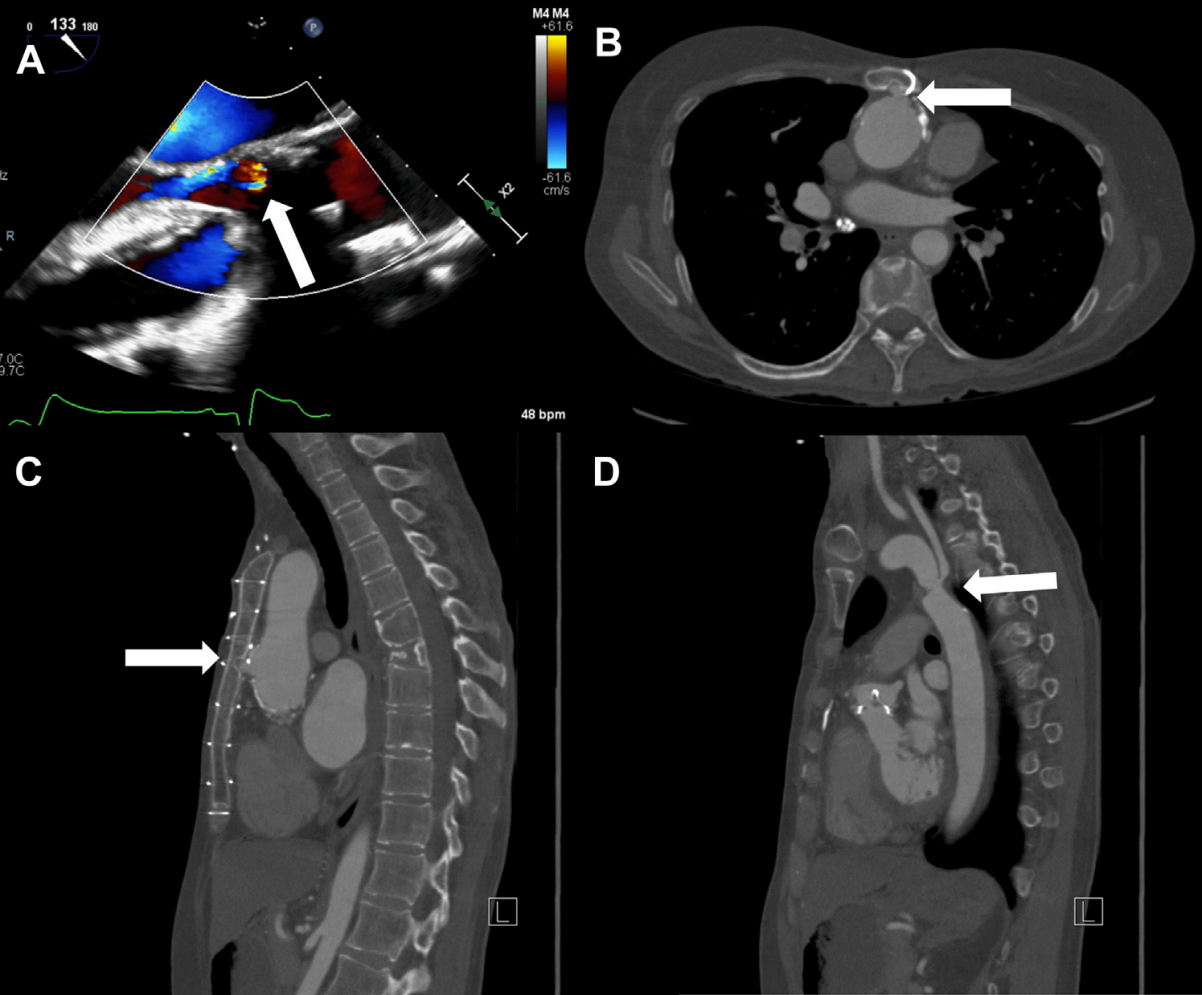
Preoperative Echocardiogram and Computed Tomography Scans Preoperative imaging with echocardiography demonstrating moderate aortic insufficiency (A). Computed tomography demonstrating an aortic pseudoaneurysm invading the sternum (B, C) and a stenotic segment of the descending thoracic aorta (D).
